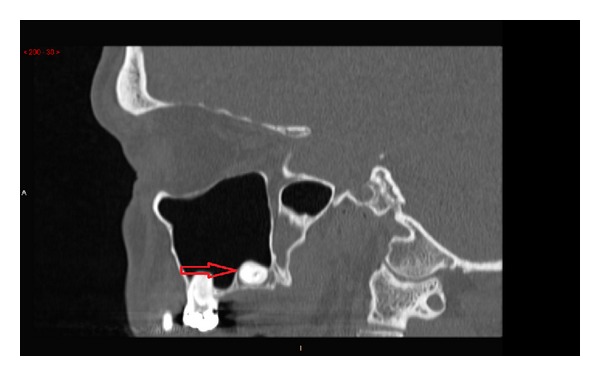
Sagittal view of the CT-scanner showing hyperdense material in the floor of the left maxillary sinus consistent with an ectopic third molar.
